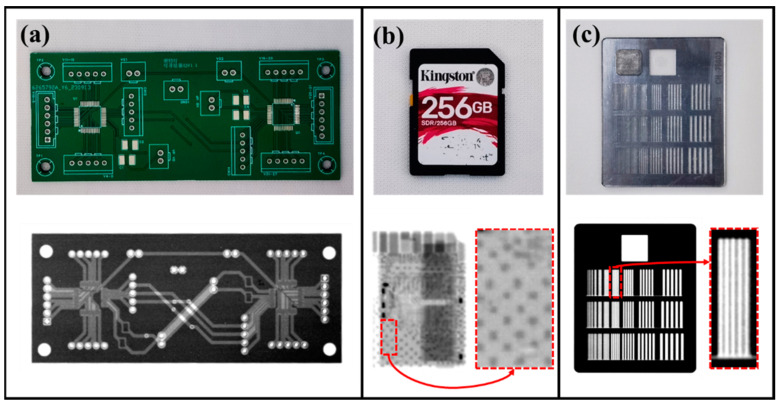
Optical (top) and corresponding X-ray (bottom) images obtained using the reflective anode FPXS. (a) PCB; (b) memory card; (c) line-pair resolution test card.
